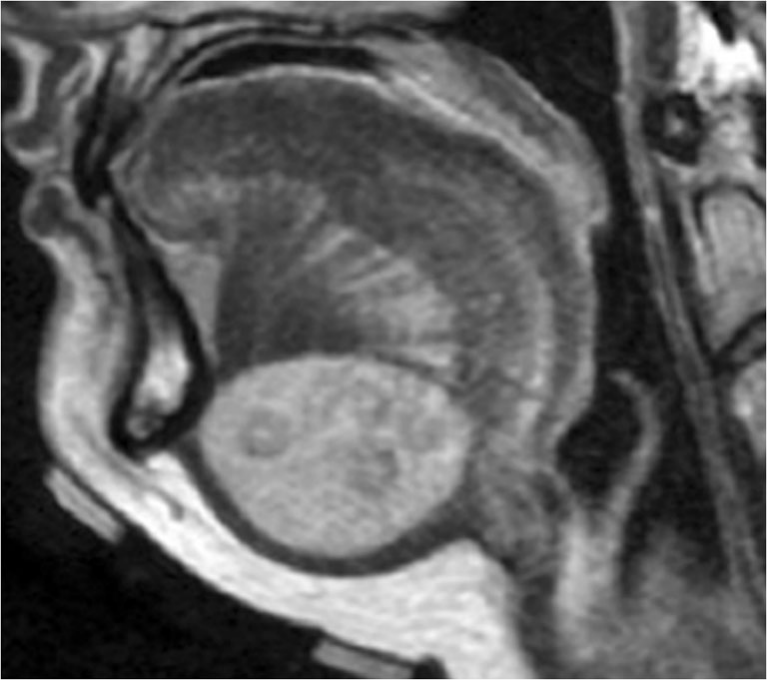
Dermoid cyst. Sagittal T2-weighted MR image shows a well-circumscribed cystic lesion filled with fat globules (“sac of marbles”)
